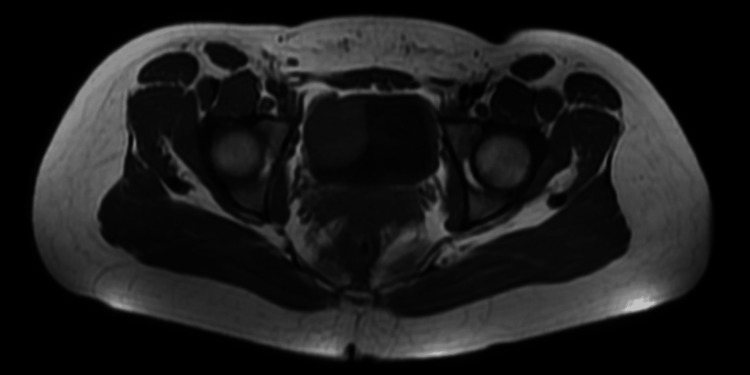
Pre-operative contrast-enhanced pelvic MRI T1 axial
